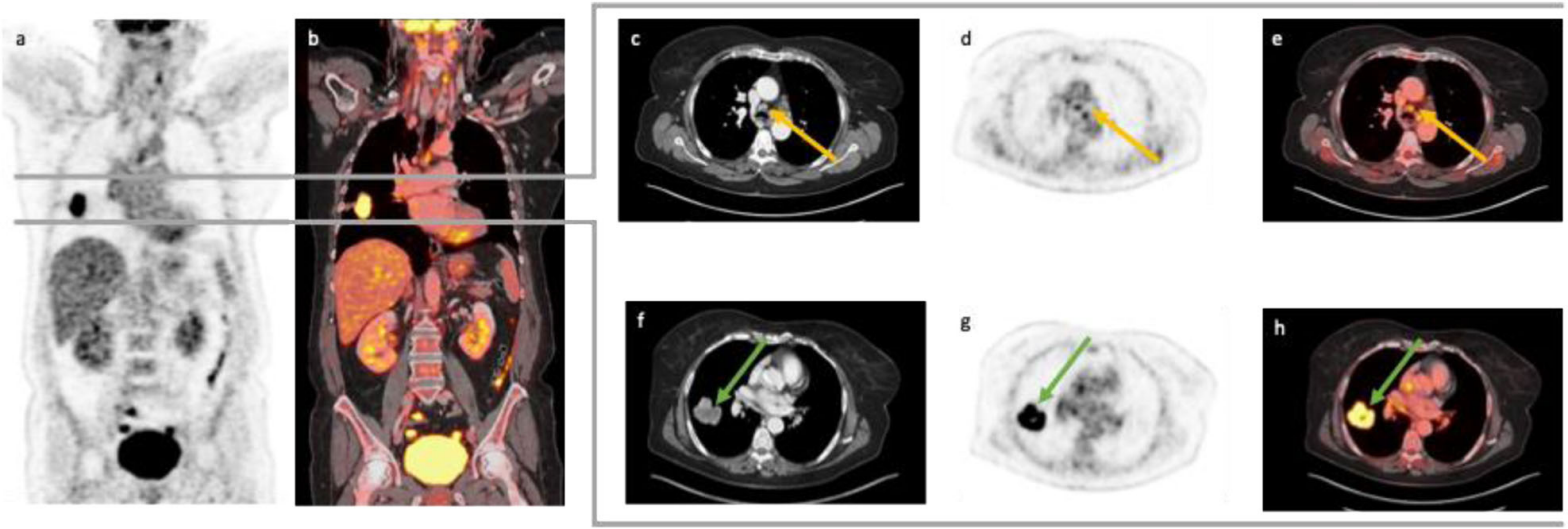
[^18^F]FDG-PET/CT (a and b) with pulmonary mass in the right upper lobe, suggestive of lung cancer. The pulmonary mass exhibited intense FDG uptake (bottom row f–h, green arrow). Several mediastinal lymph nodes were identified as pathologic on the CT scan (c). However, FDG-PET/ceCT revealed only medium FDG uptake in these lymph nodes (top row d and e, yellow arrow). A bronchoscopy was recommended for further evaluation, which confirmed no lymph node metastases in the mediastinum
